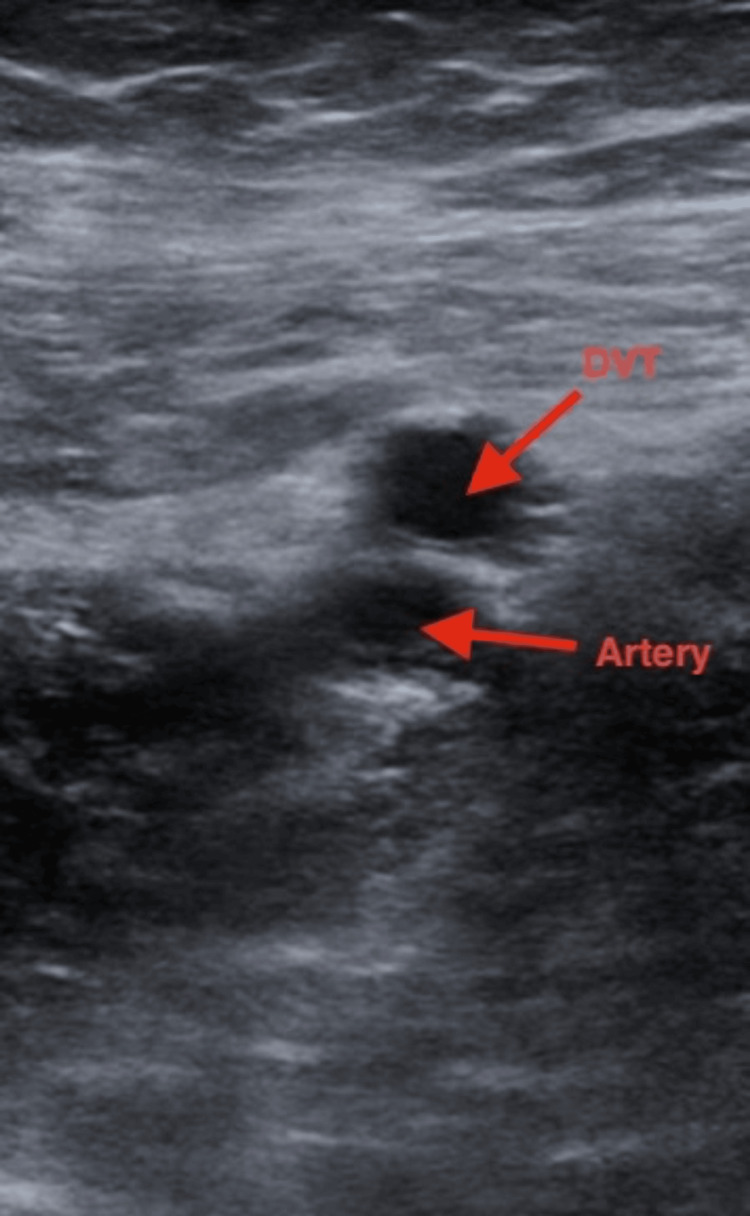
Ultrasound demonstrating right lower extremity deep vein thrombosis Transverse view demonstrating a noncompressible femoral vein containing intraluminal echogenic material (upper arrow), consistent with thrombosis. The adjacent femoral artery (lower arrow) remains patent.
